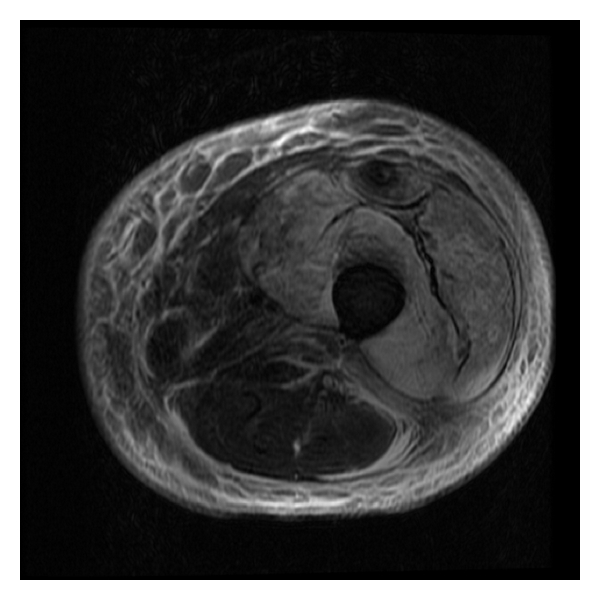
T_2_-weighted magnetic resonance image: transverse view of the left thigh: demonstrating diffuse edema in the anterior compartment and subcutaneous edema in the anterior and lateral aspects of the thigh.
